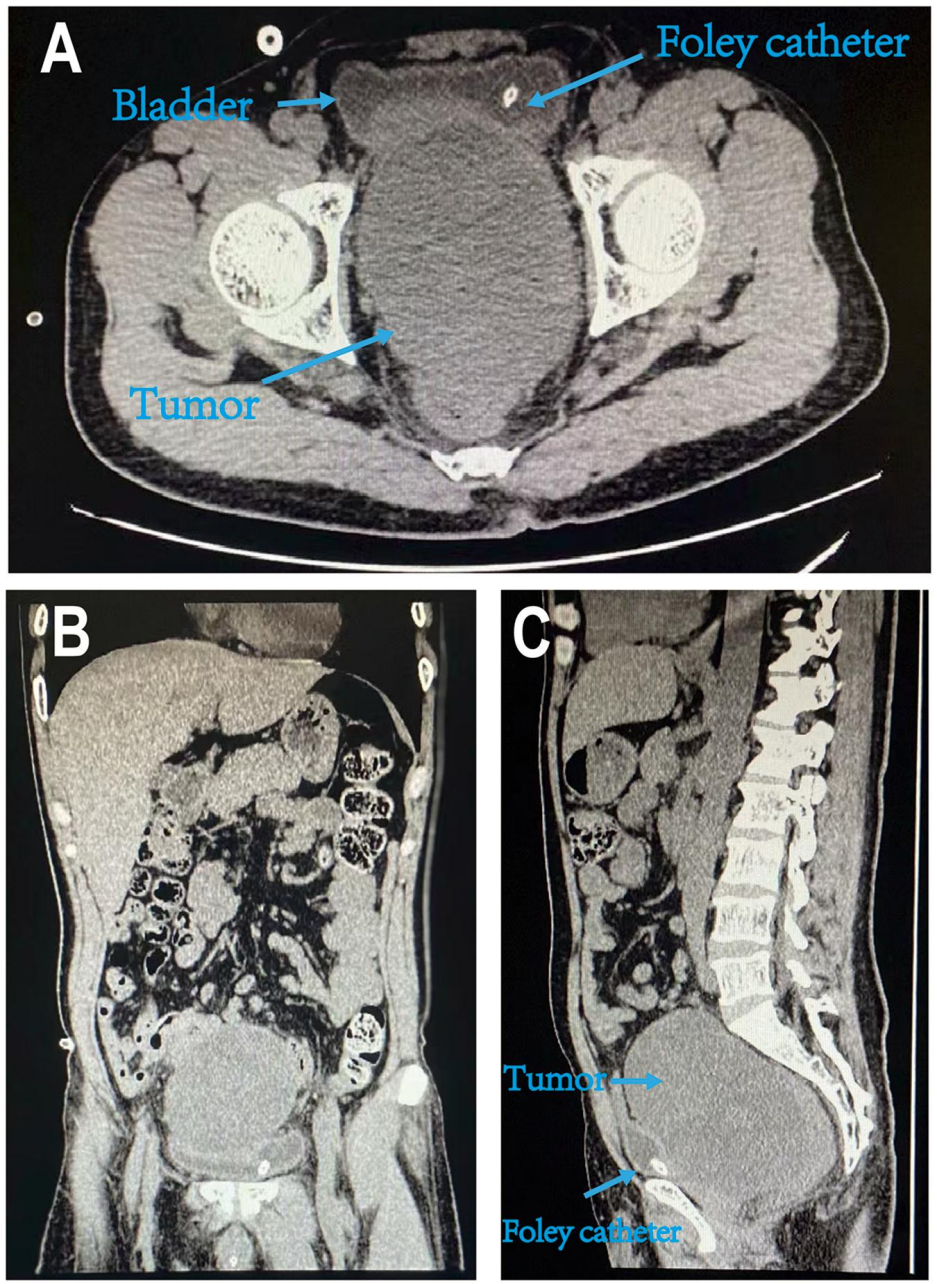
Abdominal plain CT scan image due to the sudden-onset pain and dysuria. (A–C) The axial, coronal, and sagittal CT scans revealing a round soft tissue mass located on the right side of the rectum and behind the bladder.
